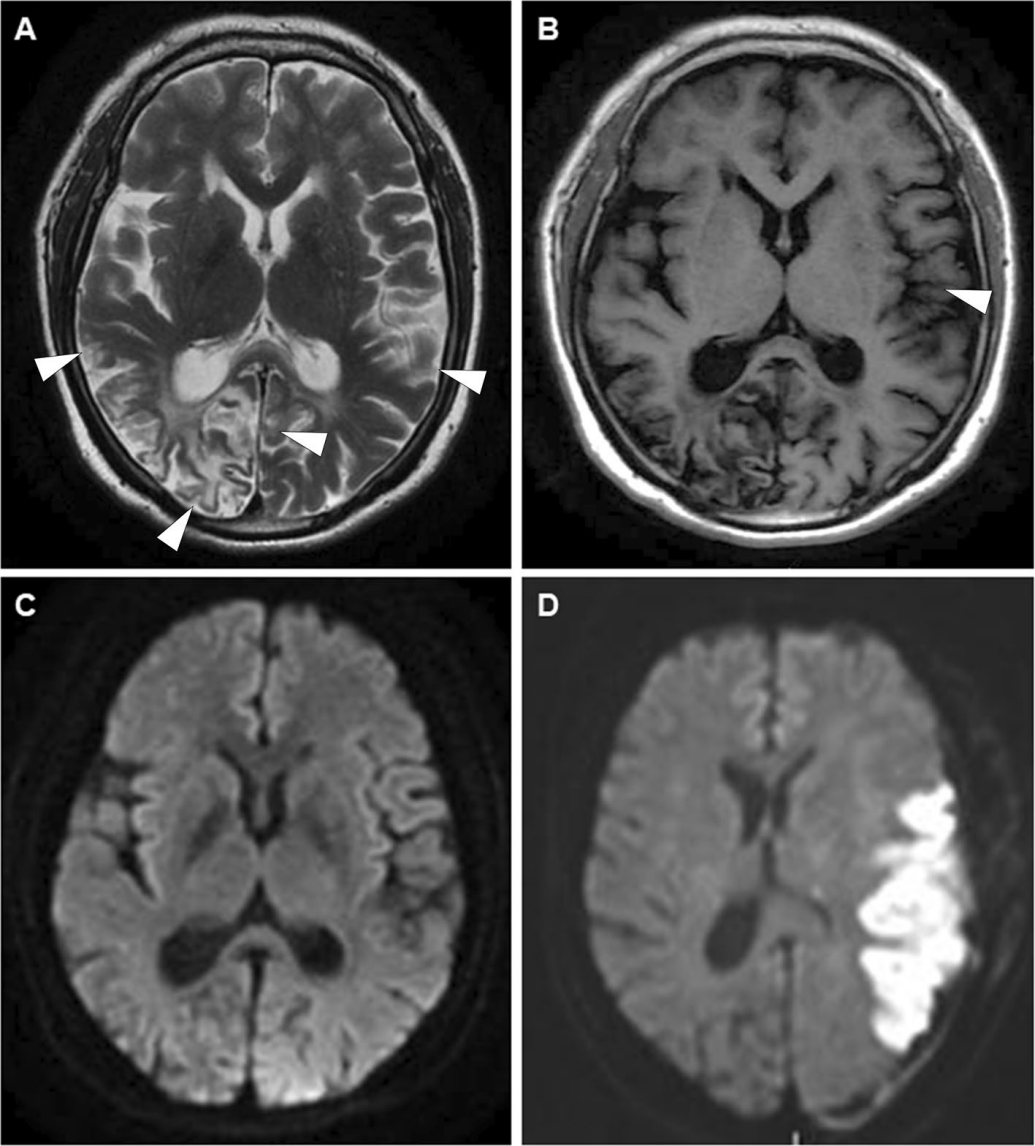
MR images obtained pre and postoperatively represent ischemic lesions. (A–C) T2-weighted, T1-weighted and diffused weighted MR images showed preoperative infarction in bilateral of temporal lobe and occipital lobe (white arrowheads). CIES = 7 point. (D) Diffused weighted MR images showed acute cerebral infarction in left temporal and occipital lobe postoperatively.
